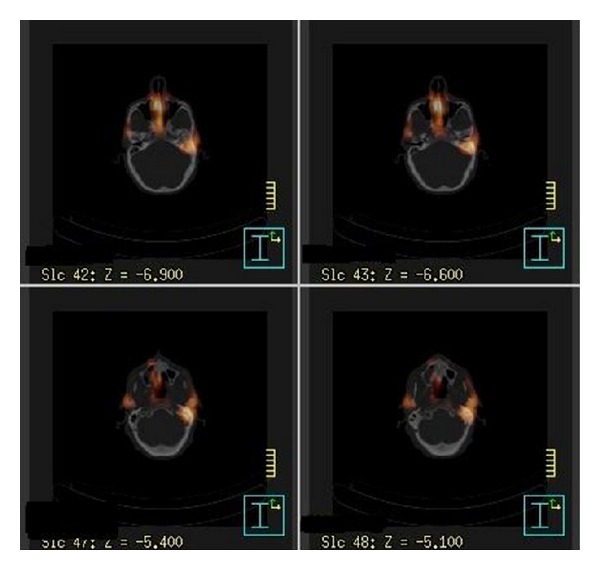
SPECT scan demonstrating increased areas of uptake in the region of the left petrous temporal bone and external auditory canal.
